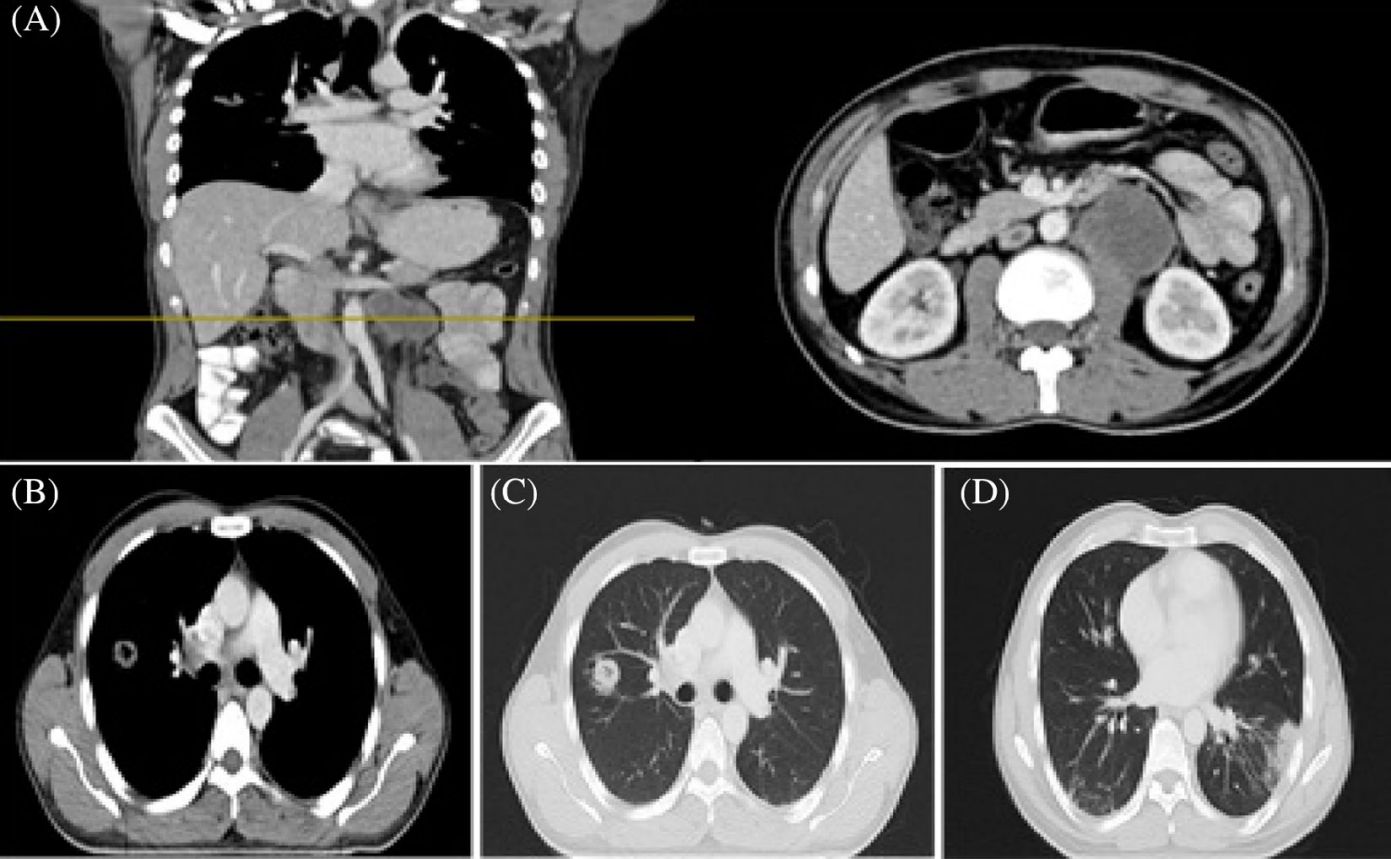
CT scan after 4 coarse BEP in December 2019. (A) Paraaortic lymph node at the level of L2–L3 (coronal and axial plane). (B) Axial plane of pathologic hilar and mediastinal lymph nodes at level of T6 (mediastinal window). (C) Axial plane of right pulmonary mass (lung window). (D) A new mass like lesion with ground glass opacity at posterior segment of left lower lobe.
